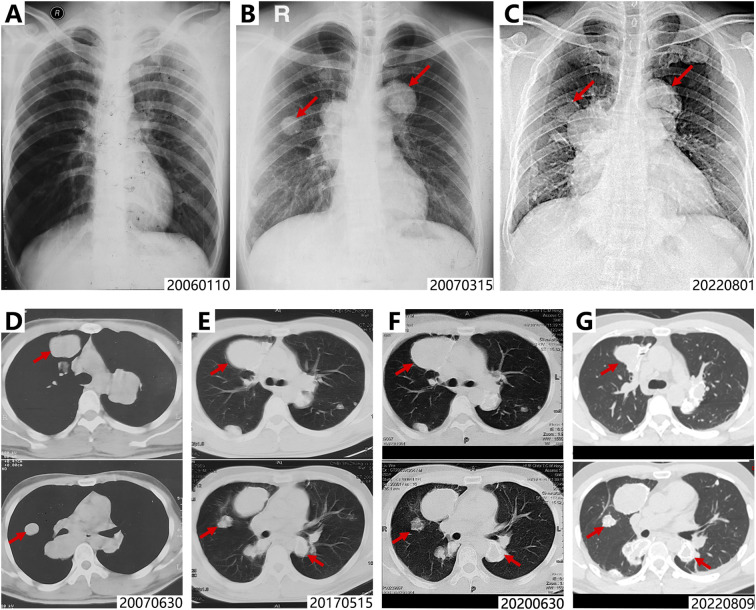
X ray and CT imaging of pulmonary metastases from Jan. 2006 to Aug. 2022. (A–C). Chest radiographs show rapid progression of pulmonary metastases (2006-2007), with gradual control achieved following chemotherapy and denosumab therapy (2007-2022). (D–G) CT imaging documented progressive tumor growth from 2007 to 2017, which was followed by disease stabilization and the emergence of characteristic peripheral rim calcification upon initiation of denosumab therapy in 2017. Red arrows denote typical changes in partial lesions.
